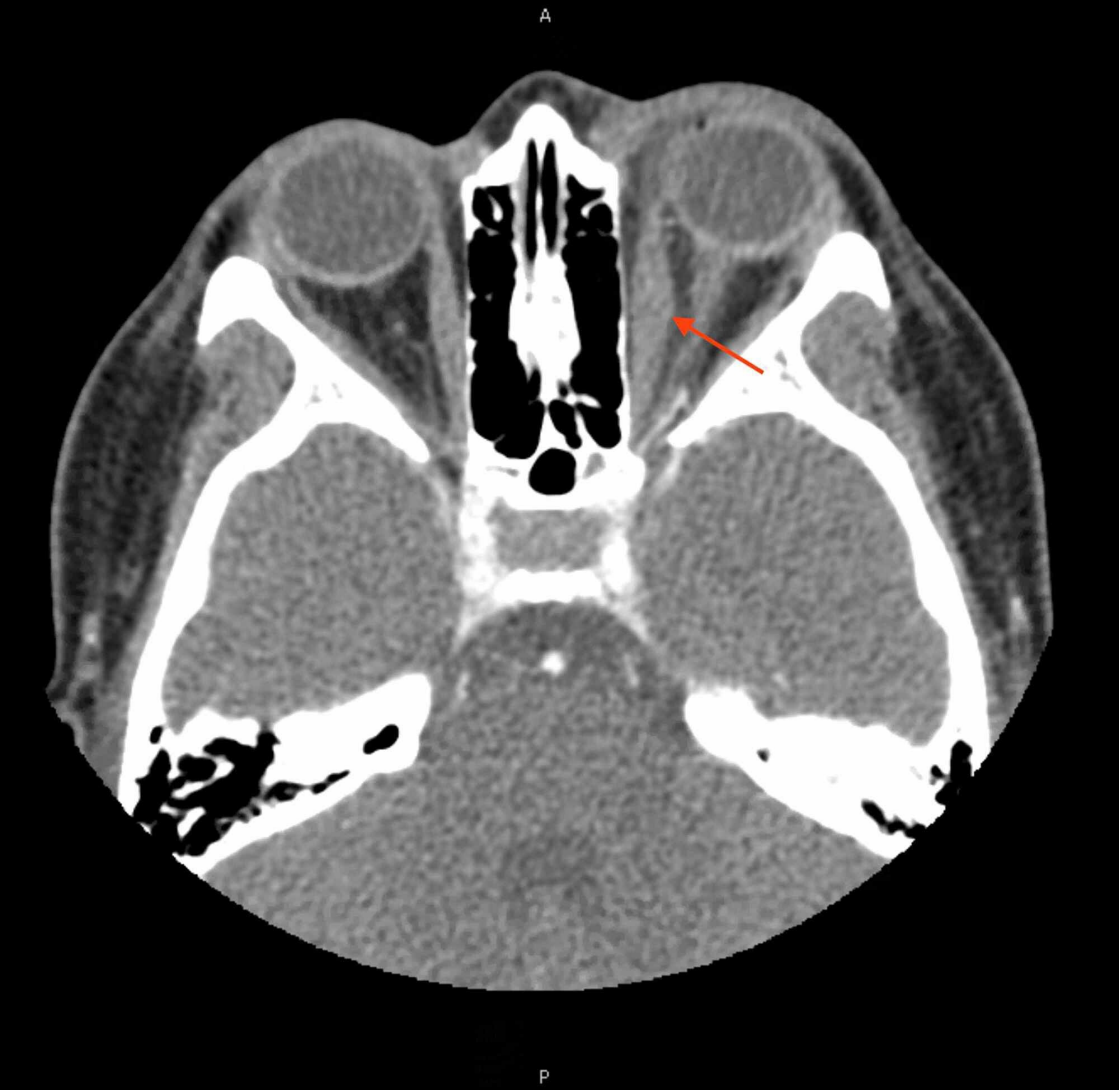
CT image with contrast showing left medial rectus swelling CT = computed tomography. Red arrow denotes the left medial rectus.
